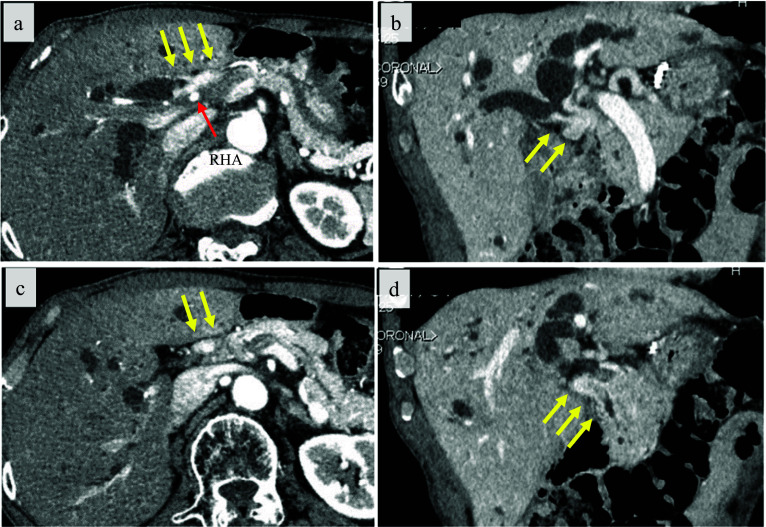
Enhanced computed tomography showed circumferential wall-thickening at the common hepatic duct (yellow arrow) with suspected invasion into the right hepatic artery (RHA; red arrow)
